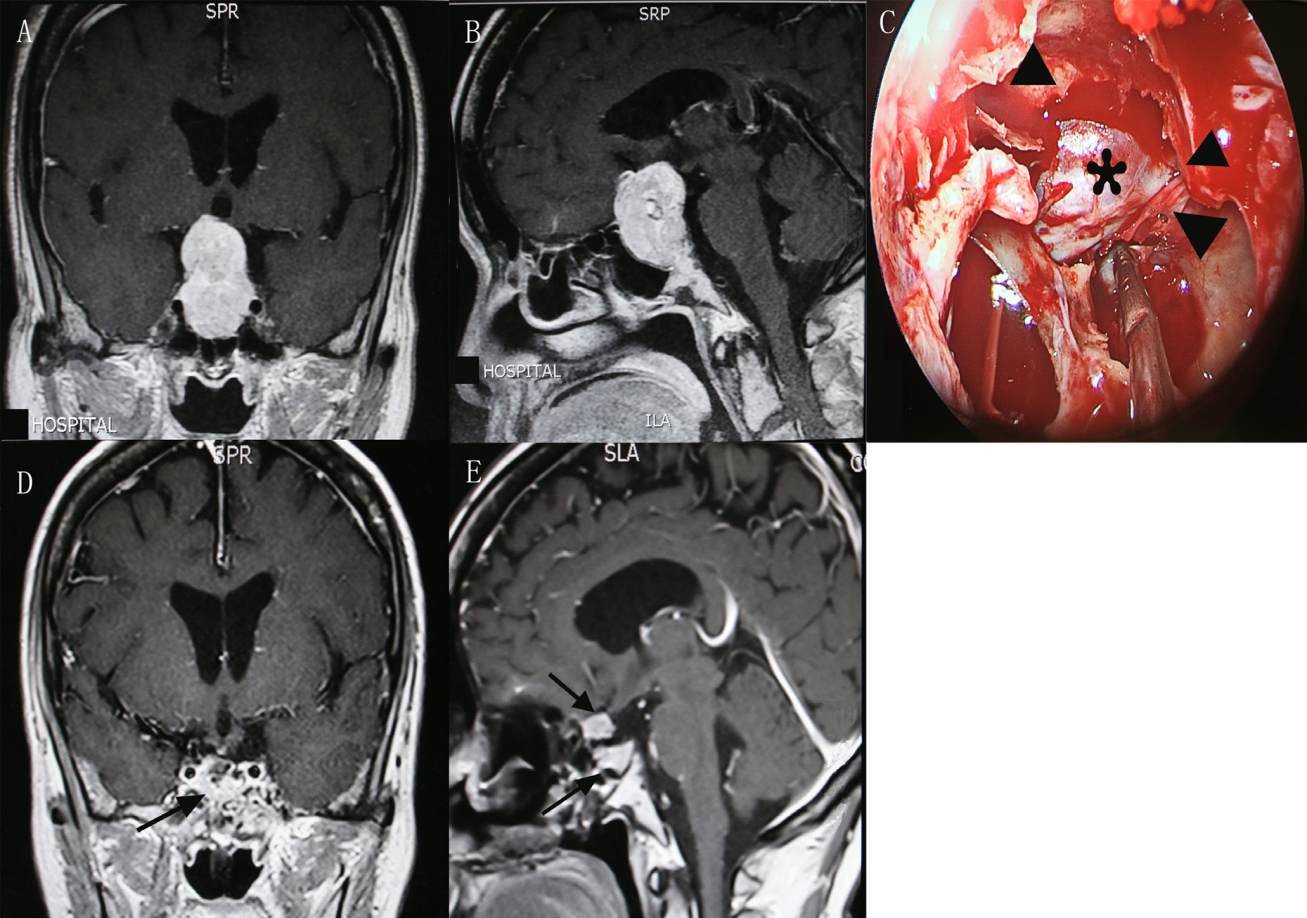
Contrast-enhanced MRI demonstrated preoperative coronal (A) and sagittal (B) views of a giant pituitary adenoma. The suprasellar adenoma (asterisk) was viewed under direct visualization after the opening of diaphragma sellae (triangle) from the endoscopy. (C) Postoperative coronal (D) and sagittal (E) views showed a small residual of tumor and fat graft (arrow) placed in the pituitary fossa. MRI, magnetic resonance imaging.
